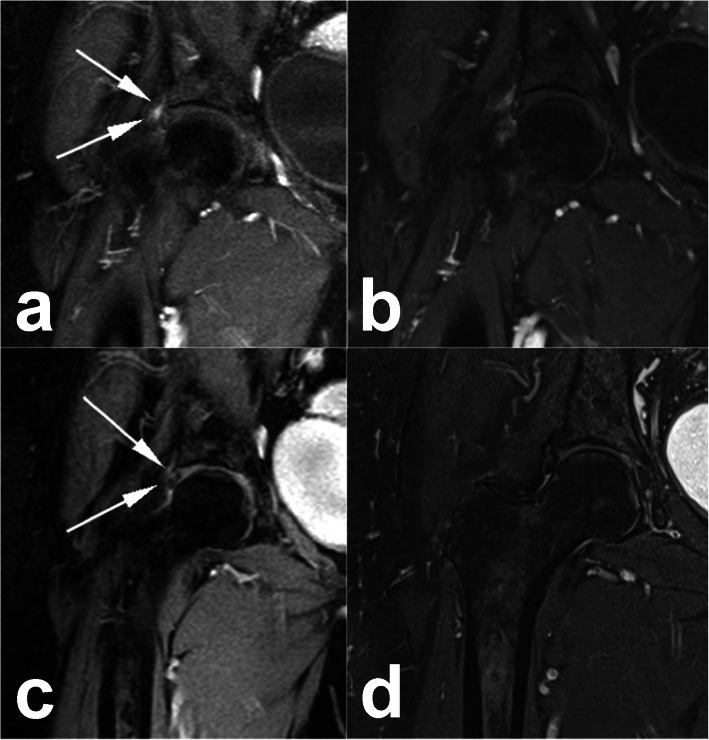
Coronal Magnetic Resonance image of the right hip joint. a and b pre and post embolization DPW-weighted image with adipose tissue saturation. c and d pre and post embolization Post-contrast T1W-weighted image with tissue saturation. In (a and c) note a degeneration in the superolateral segment of the acetabular labrum with mild pericapsular edema and post-contrast enhancement (arrows). In the four- month post-procedure image, the pericapsular edema was almost completely resolved (b), and a partial resolution of post-pericapsular contrast enhancement (d)
